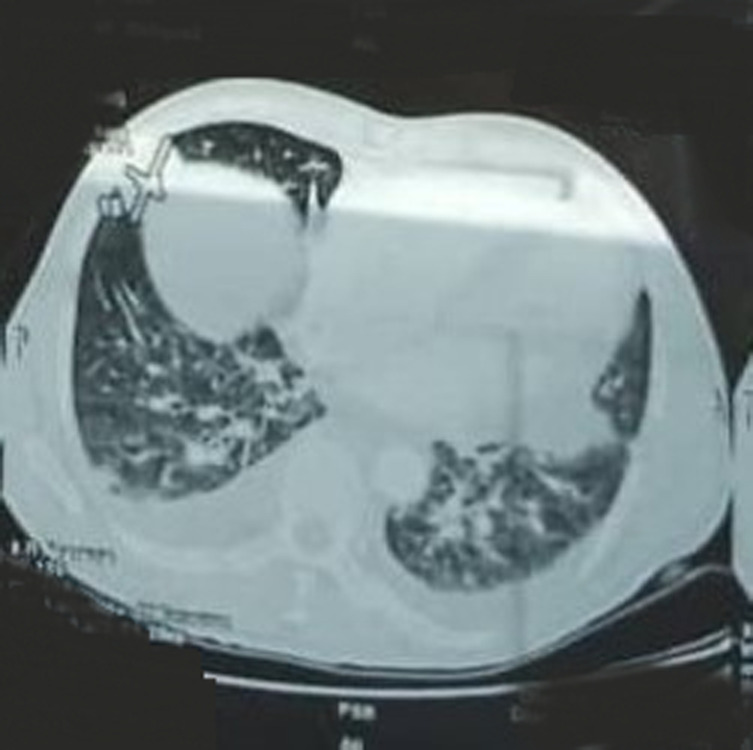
thoracic stage of a CT scan revealed multiple nodules at the upper lobar and bilateral lingular level with a medium lobar macronodule of 39 mm × 18 mm compatible with secondary pulmonary localizations
